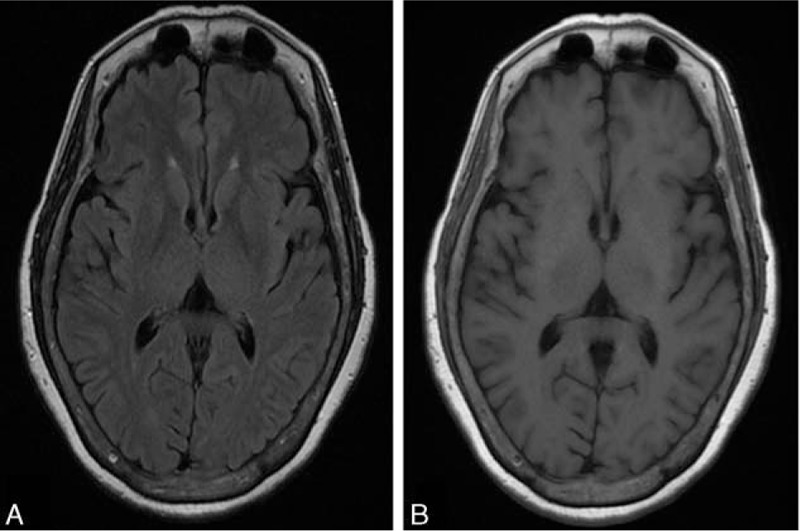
Brain magnetic resonance imaging of Case 2 showing normal appearing brain parenchyma, which was disproportionate to her cognitive deficits (A) T2 fluid-attenuated inversion recovery image, axial view. (B) T1-weighted image, axial view.
